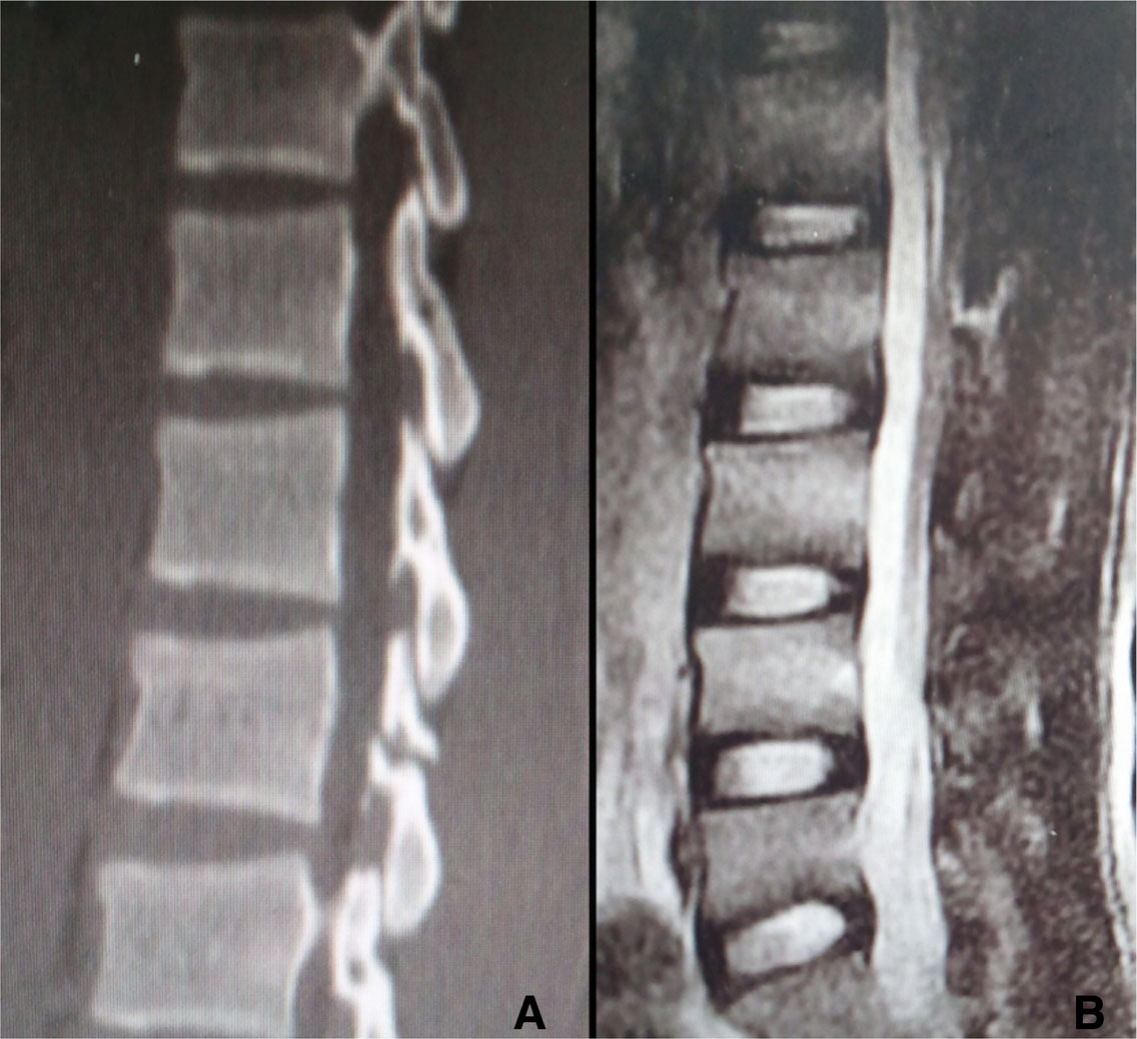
a Sagittal CT scan and b sagittal MRI showed L2 flextion-compression fracture
